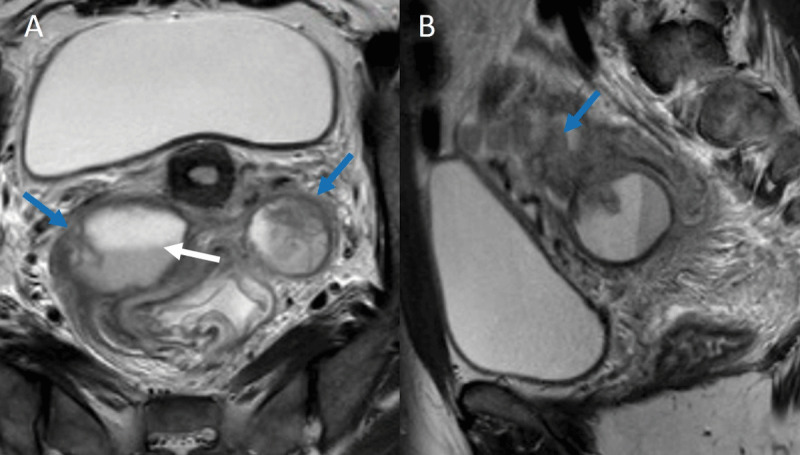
A week later, the patient presented to the emergency room while under antibiotherapy for persistent pelvic pain. A computed tomography (CT) scan was performed and revealed a persistent tubo-ovarian abscess with extension to the recto-uterine pouch (Figure 3A, white arrow). Laparoscopy confirmed the diagnosis (Figure 3B, white arrow).
